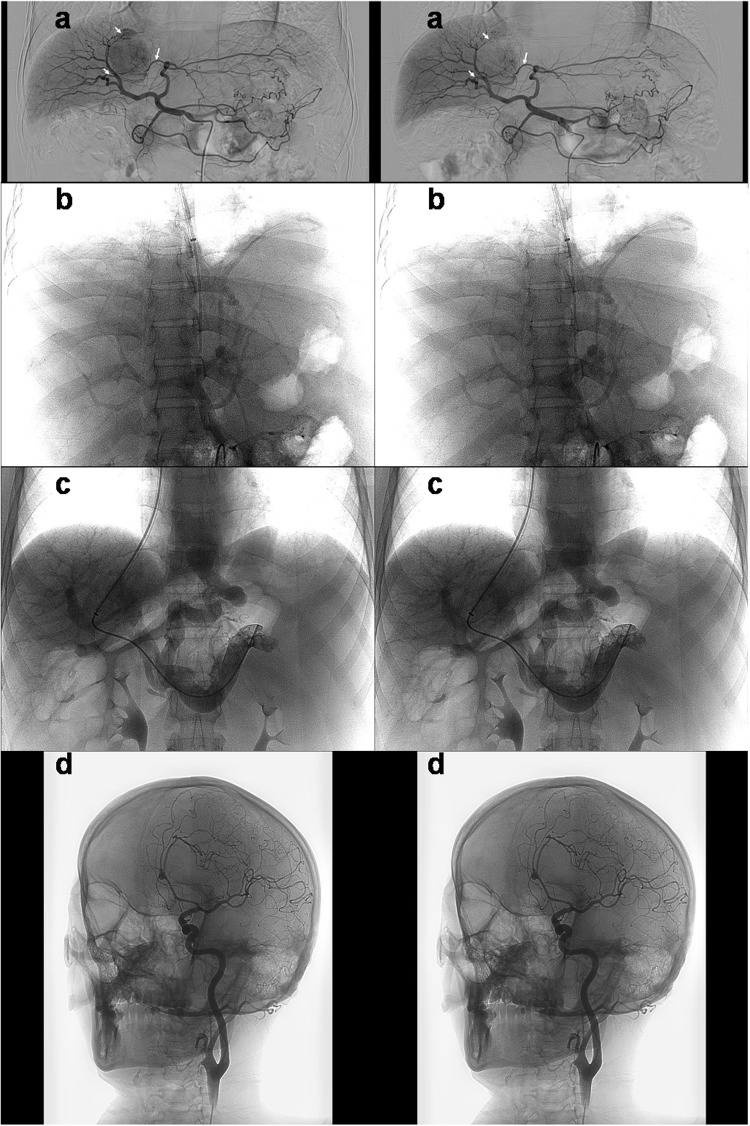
(a) Duplicate plain X-ray images from a patient with hepatic cancer during TACE were horizontally combined. The tumor’s supply vessels and the anteroposterior direction of overlapping vessels were clearly identified (the white arrows). Duplicate plain X-ray images of direct portography (b), indirect portography (c) and internal carotid angiography (d) with different α angles (RAO −16.0°/−18.0°, LAO 8.0°/10.0°, and LAO 22.8°/24.6°) were horizontally combined. Observation with VR glasses produced realistic stereo images of structures such as vessels, bones, angiographic guidewires and catheters.
